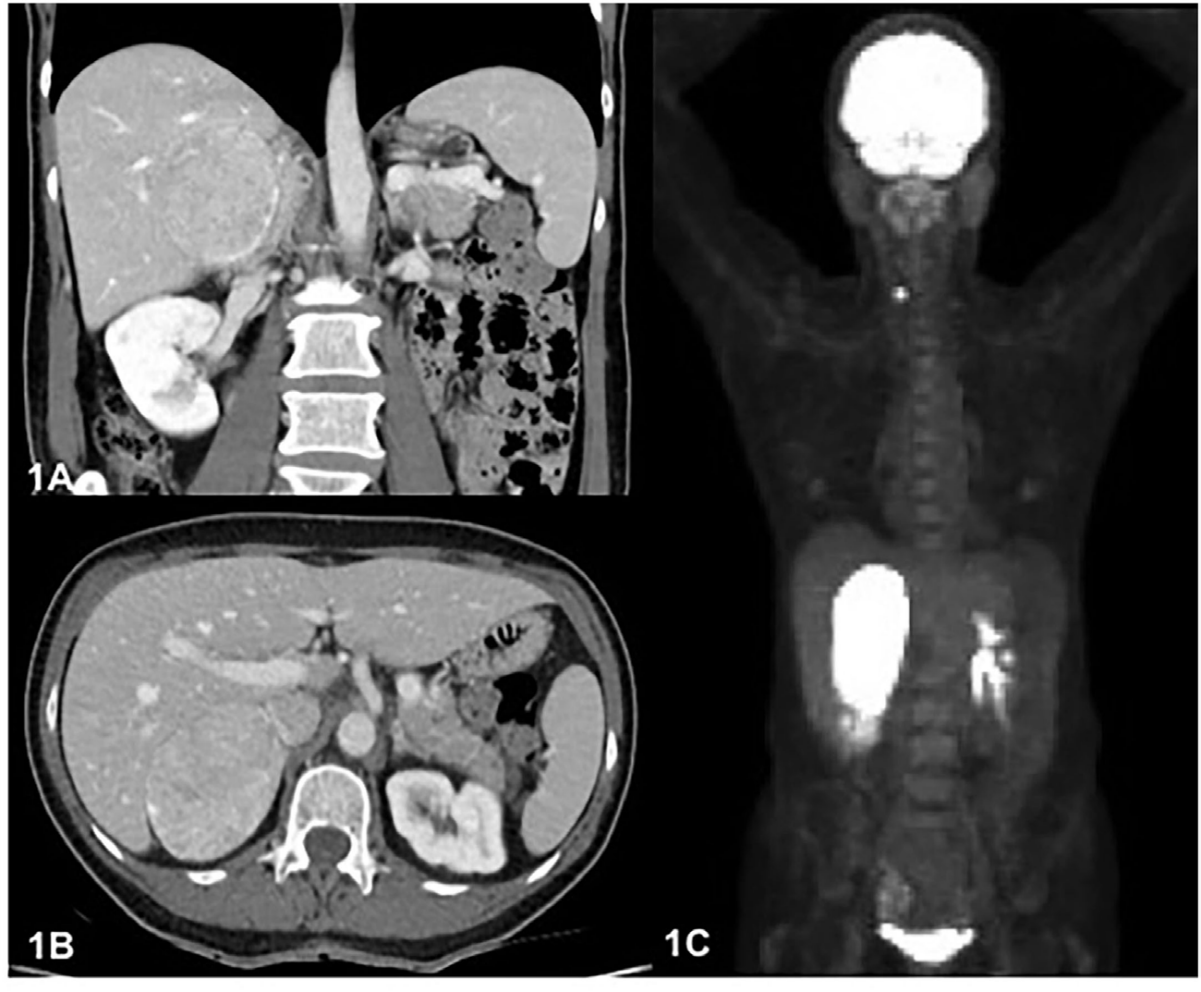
(A). Axial image on portal phase computed tomography (CT) scan showing right adrenal mass heterogeneously enhanced of 7.6 cm × 5.8 cm. (B). Cross-sectional image on CT scan showing right adrenal mass with imprint on the liver. (C). Maximum intensity projection image on FDG positron emission tomography showing the two hypermetabolic masses, right adrenal, and right thyroid lobe.
